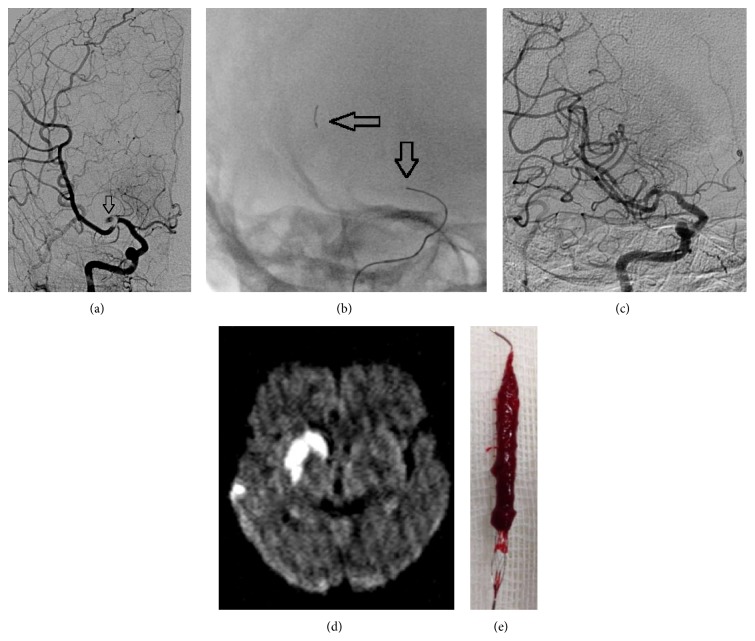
(a) Angiogram shows a thrombus on proximal MCA and an occlusion of superior division of MCA. (b) Deployed REVİVE device (distal and proximal markers = black arrow). (c) After retrieval of the stent, the vessel is recanalized to a TICI-3 state. (d) Control MRI scan shows right hemispheric striatocapsular infarction and multiple parietal small embolic infarctions. At 3 months, patients had a good outcome. (e) Large thrombus is adherent to the stent struts.
